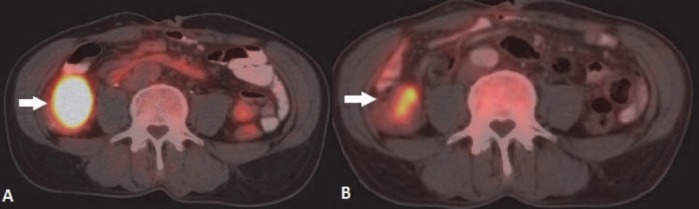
NHL of right colon. FDG avid circumferential mural thickening of caecum near the iliocaecal junction is seen (A). Post therapy scan (B) show partial response
